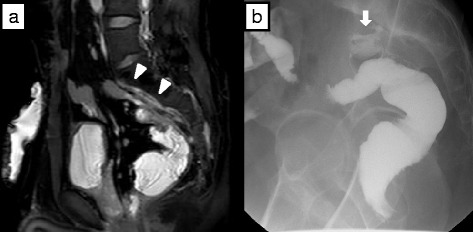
a T2-weighted MR image reveals soft-tissue intensity with enhancement anterior to S1–S3 (*white arrowheads*). b Lateral radiograph during Gastrografin enema shows extravasation of contrast material from the top of the pouch to the S1–S2 presacral space (*white arrow*), indicating the existence of residual abscess. There is no communication between the abscess and epidural space
